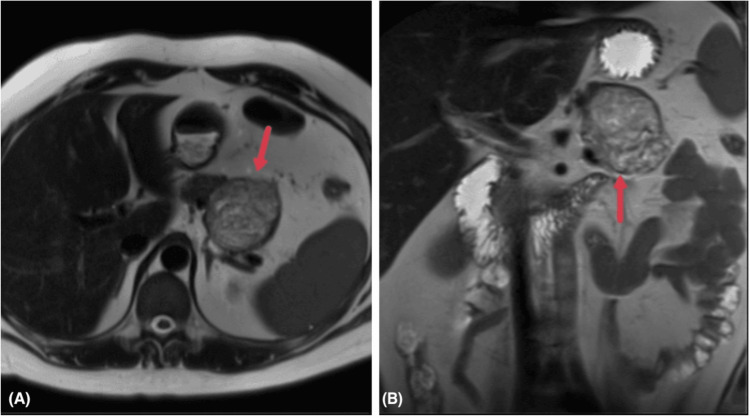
MRI of the abdomen: axial T2W (A) and coronal T2W (B) images showing the well-defined mass arising from the pancreatic tail with a heterogeneous intermediate-to-high T2 signal with a hypointense capsule (red arrows).
